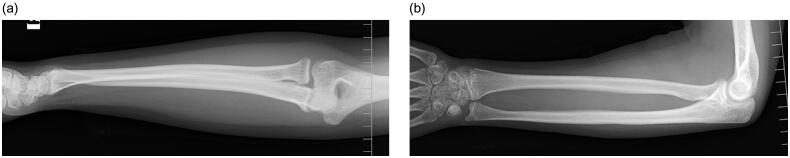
Anteroposterior (a) and lateral (b) views of radiographs 4 years after surgery.
